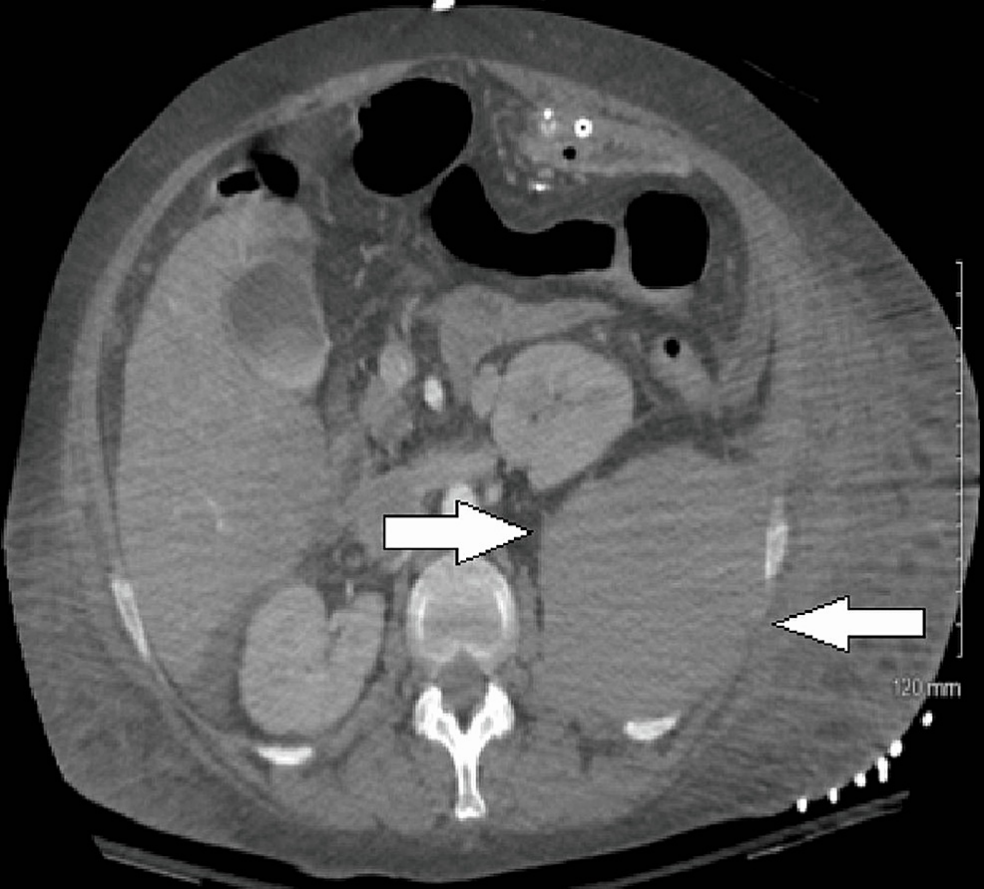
CT image showing spontaneous left retroperitoneal hematoma (arrows)
